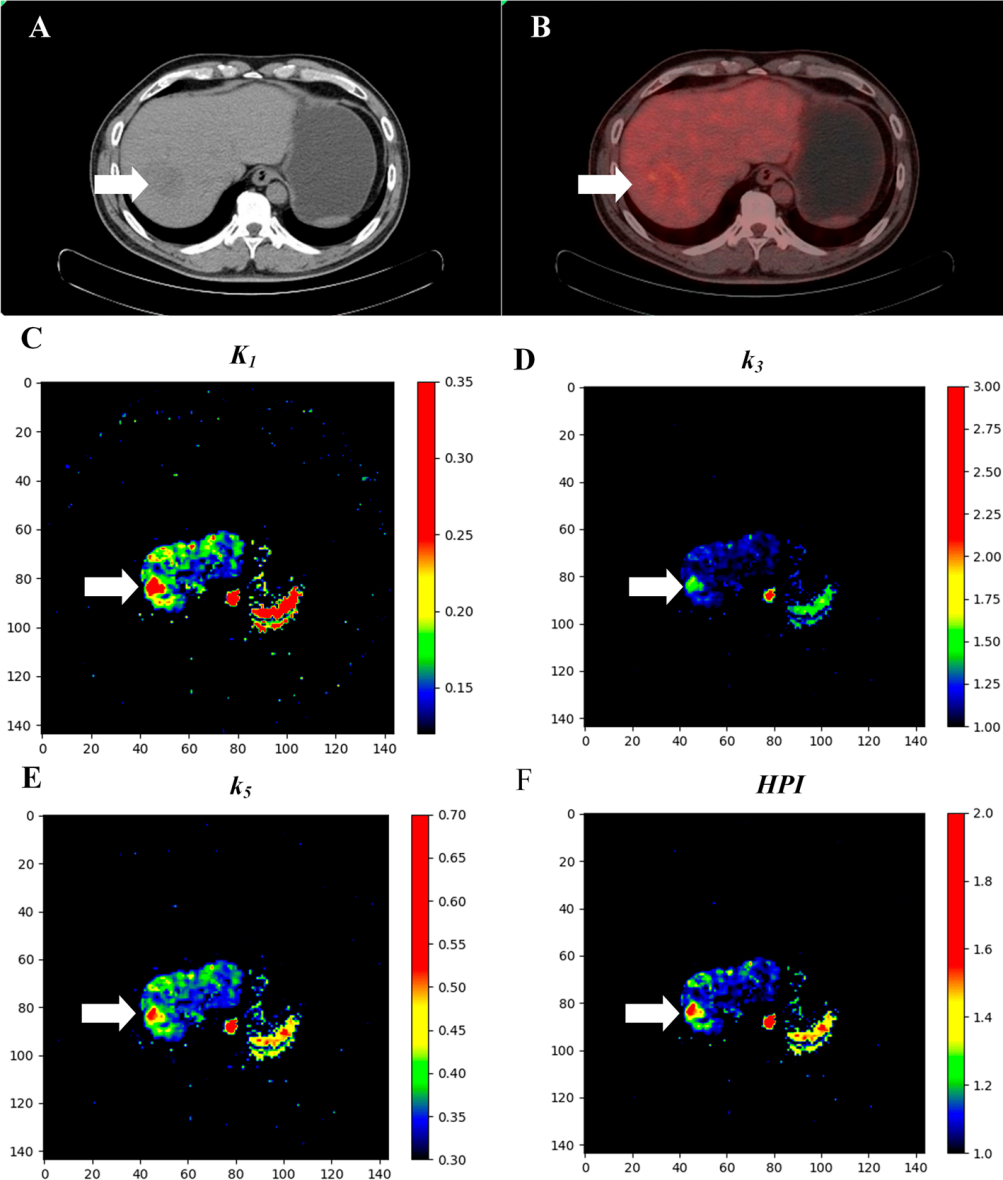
The parametric images from the 4C model show hepatocellular carcinoma (arrow) with negative findings on conventional metabolic PET/CT. A CT image. B Conventional static PET/CT image. C Parameter image of *K*_1_. D Parameter image of *k*_*3*_. E Parameter image of *k*_5_. F Parameter image of *HPI*
